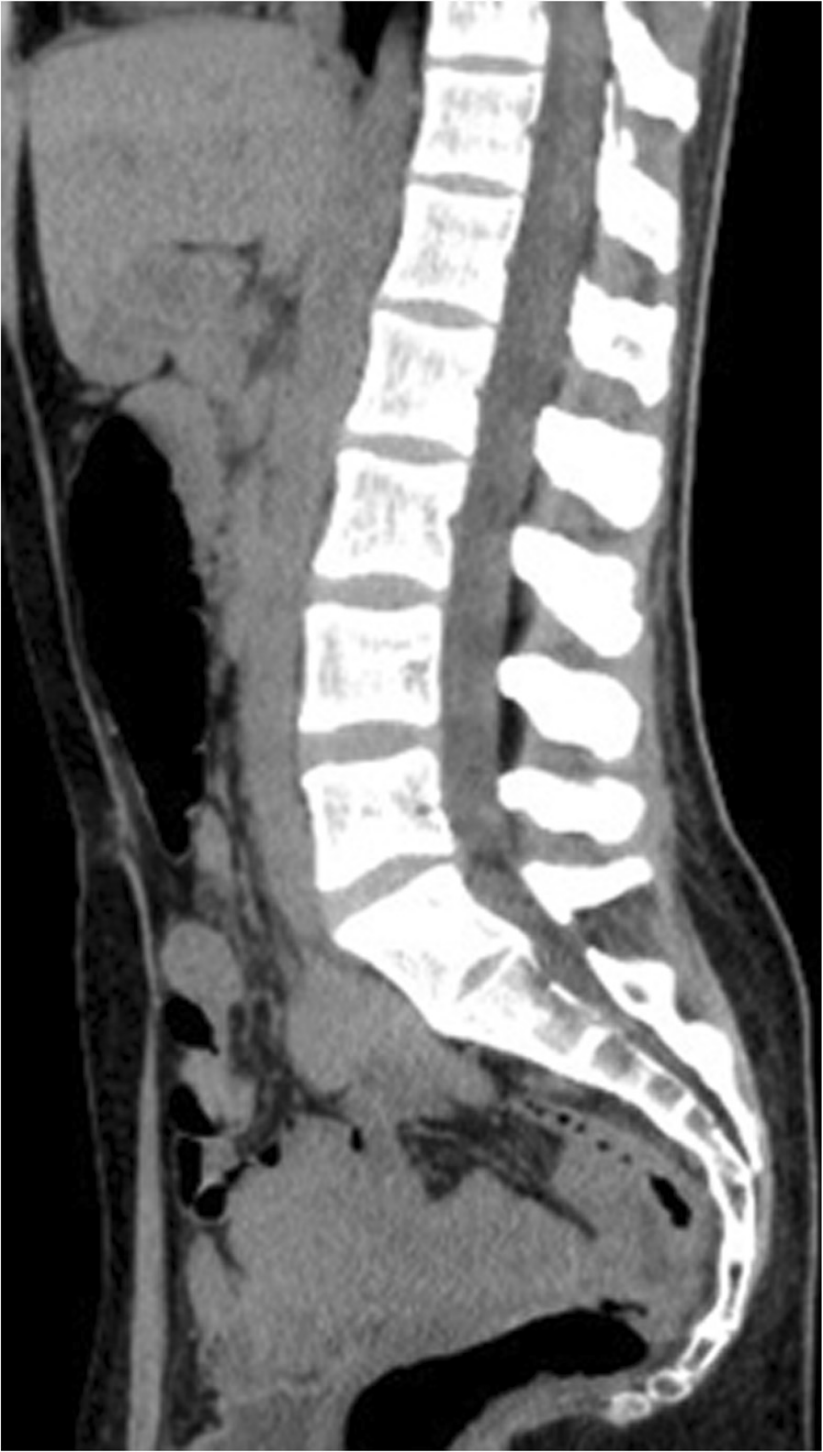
Abdominal and pelvic sagittal computed tomography (CT) image on disease day 2. CT showed no abnormalities
